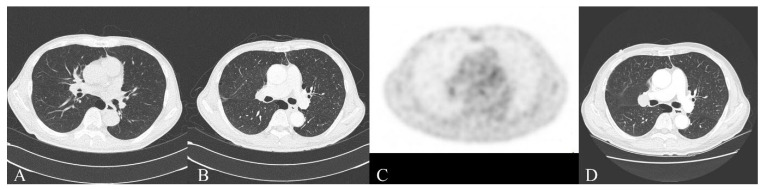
The first CT showed emphysema, and the final CT showed an enlarged LN in the right interlobar nodal station. (A) Chest CT at the time of diagnosis with LEMS; (B) chest CT 9 months after the diagnosis of LEMS; (C) PET CT 9 months after the diagnosis of LEMS; and (D) chest CT 21 months after the diagnosis of LEMS, allowing for a diagnosis of lung cancer.
